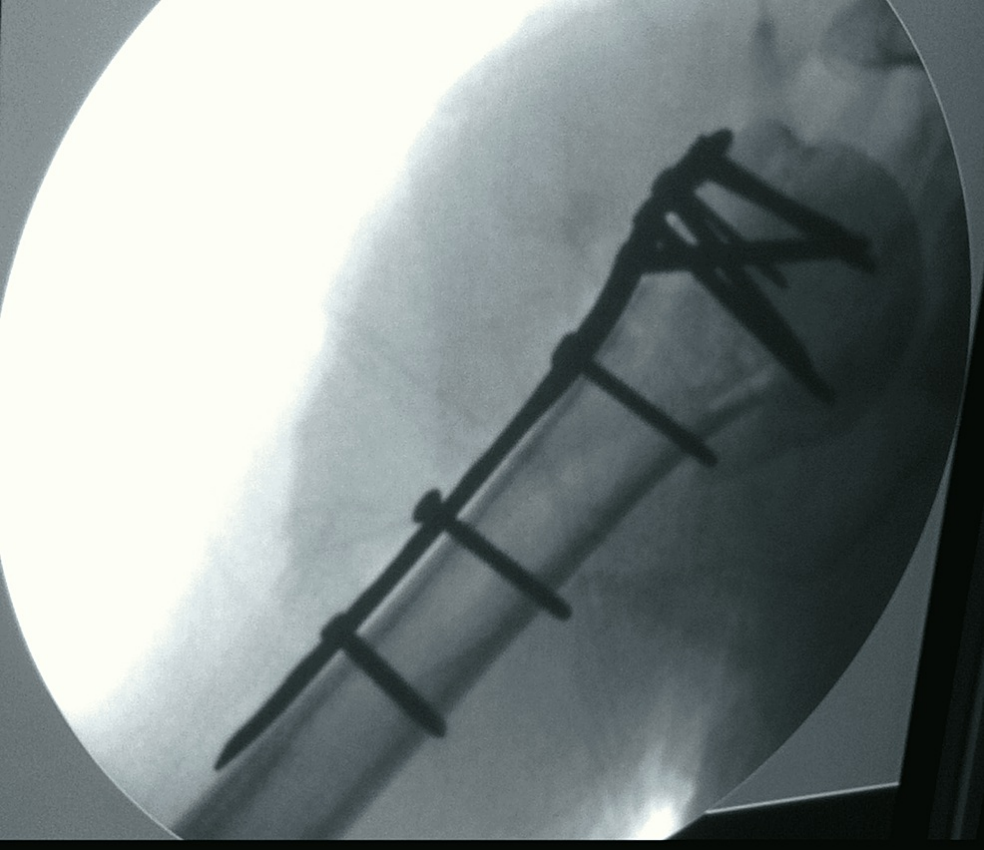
Intra-operative radiograph showing AP view of applied PHILOS plate AP view: Anteroposterior view; PHILOS: Proximal Humeral Interlocking System
